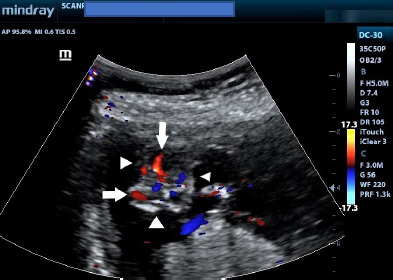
Axial Colour Doppler ultrasound images of the head showing the absence of the calvaria. Note the dysplastic vessels (white arrows) within the brain. The arrowheads indicate the margins of the bare brain
